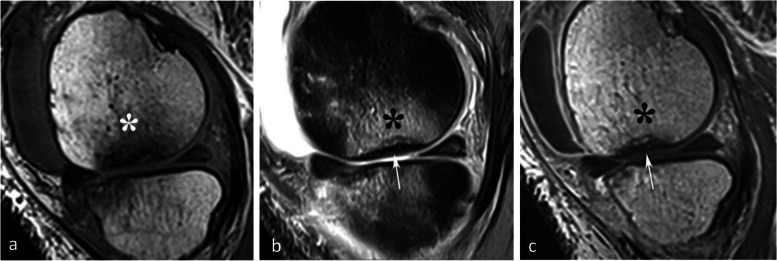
Enhancement pattern of a presumed subchondral insufficiency fracture with osteonecrosis. a T1-weighted MR image shows low signal intensity predominating near the condylar surface, extending deep in the epiphysis, and gradually attenuating with blurred outlines (asterisk). b, c On fat-suppressed T2-weighted image (b), this area (asterisk) shows high signal intensity and enhances after intravenous contrast injection (asterisk on post-contrast T1-weighted sequence in c). In this case, the subchondral area immediately adjacent to the bone plate (arrows) shows low signal intensity with fat-suppressed T2-weighting (in b) and no enhancement with post-contrast T1-weighting (in c). This pattern corresponds to an area of degraded subchondral fatty marrow, suggestive of a SIF-ON
